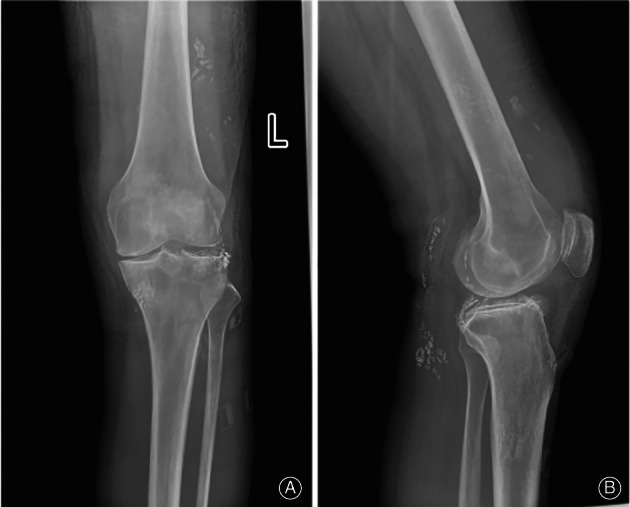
The anteroposterior (A) and lateral digital (B) radiography of the left knee. The medial space of the left knee joint narrowed, and the edges of the articular surface showed different degrees of bone hyperplasia, and the intercondylar bulge mutations. Multiple spot‐like high‐density shadows can be seen around.
